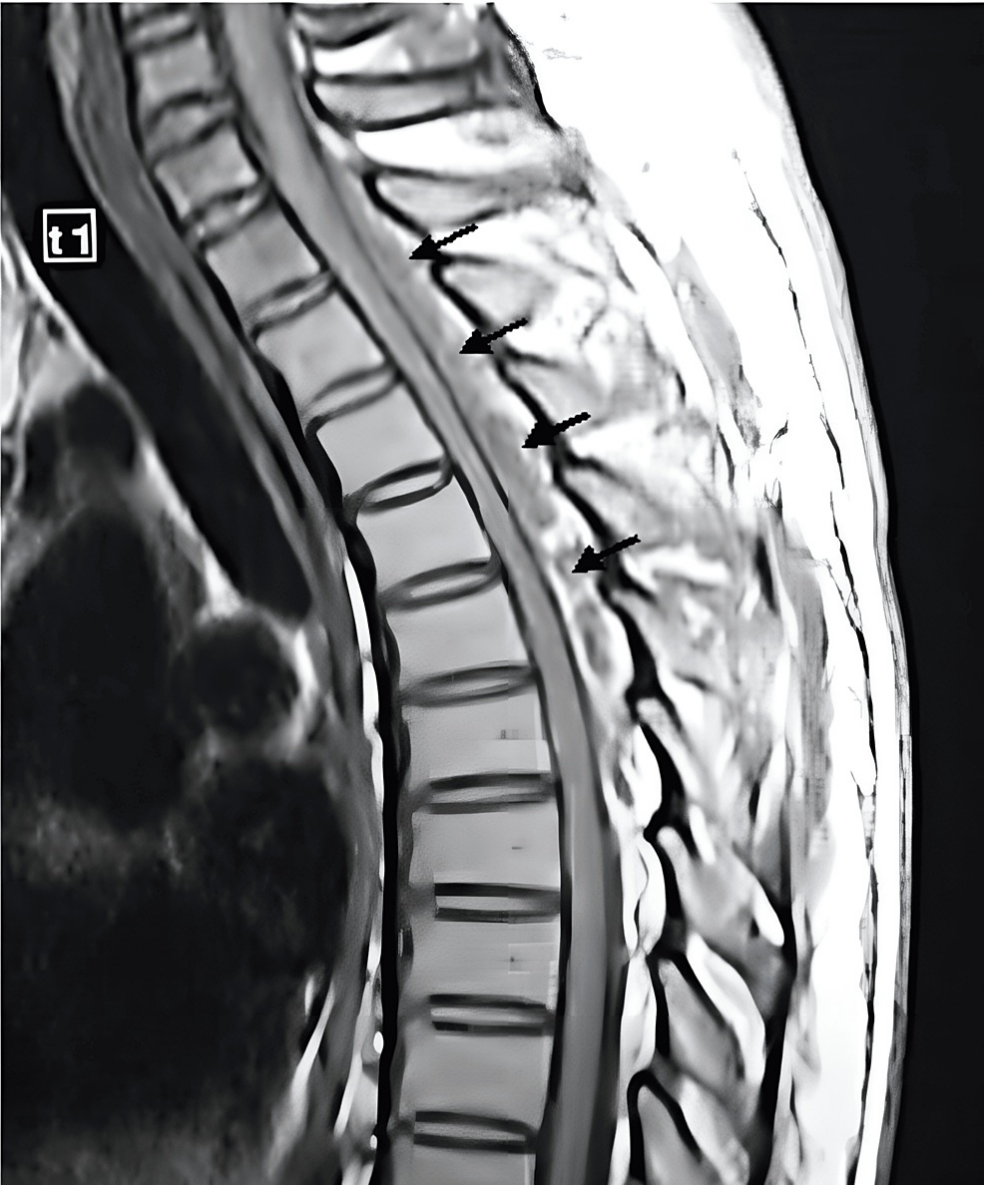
Sagittal T1-weighted MRI The image demonstrates a poorly defined and heterogeneous cervicothoracic spinal epidural abscess at C6-T10. There are hypointense and isointense images in the posterior region of the spinal canal MRI: magnetic resonance imaging
